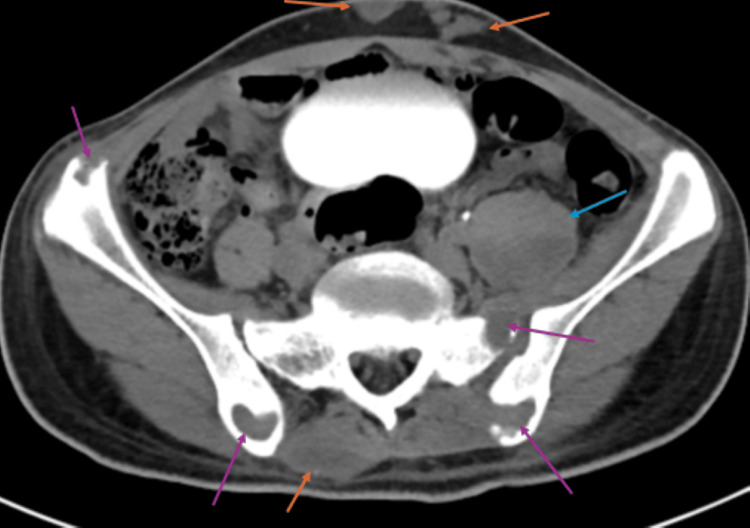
Non-contrast enhanced pelvic CT (with contrast in the urinary tract from a prior exam) demonstrating bilateral iliac destructive bone lucencies due to osteomyelitis, as well as a left sacral destructive lesion also involving the sacroiliac joint (purple arrows); left iliopsoas enlargement due to infection (blue arrow); and subcutaneous infectious lesions (orange arrows) CT: computed tomography
